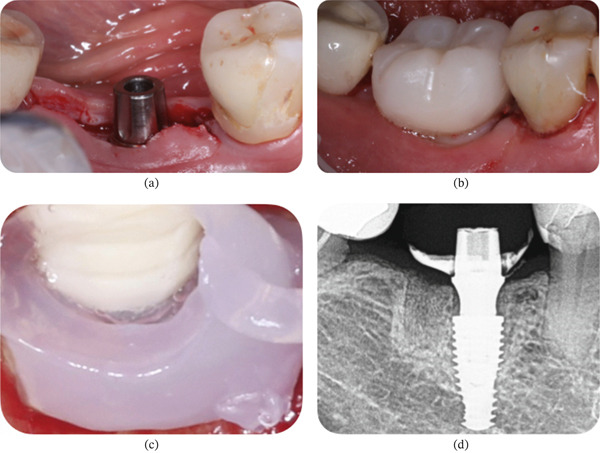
Patient #2 (right side). (a) Lateral view after the delivery of the abutment (Universal Click GM Exact). (b) Close‐up view of the surgical site after application of oral gel with active oxygen (BlueM) before closing the flap. (c) Lateral view after delivery of the provisional restoration. (d) Periapical radiograph taken immediately after the procedure.
